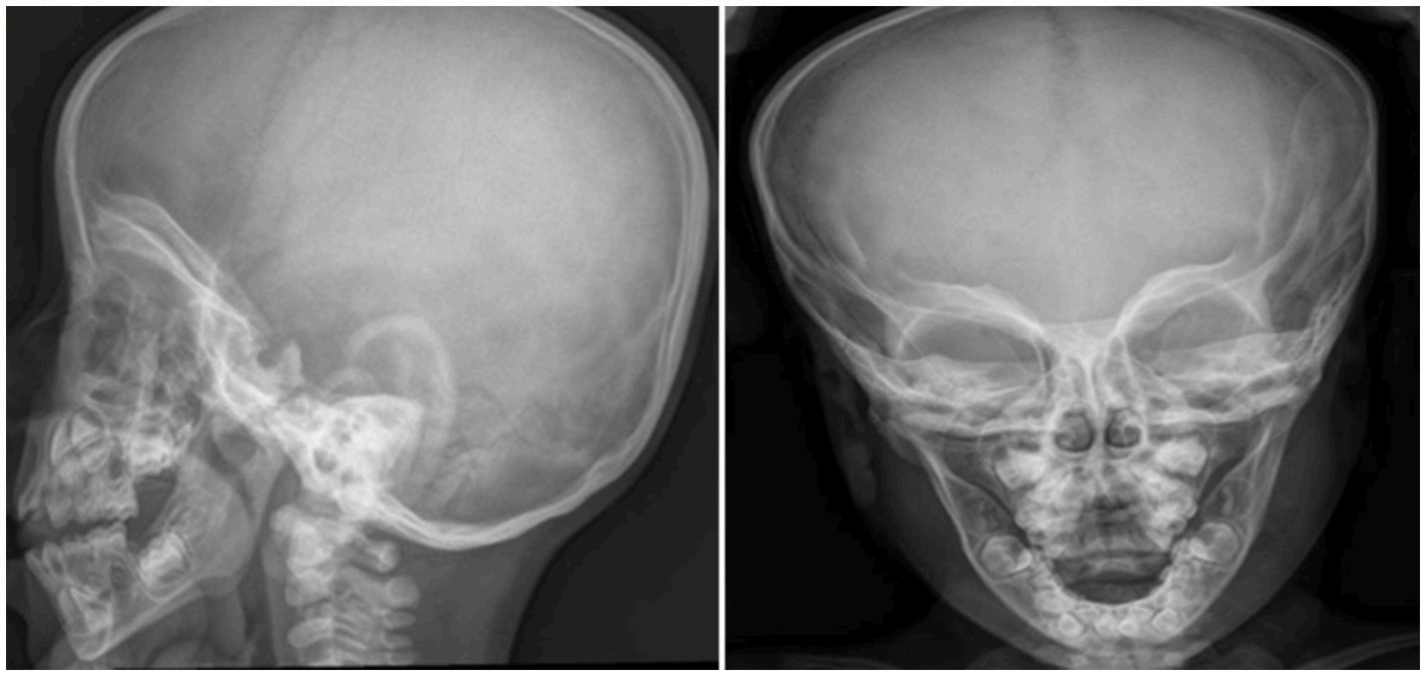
Cranial x-ray shows increased biparietal diameter, frontal bossing, “harlequin mask apparence” of orbits with increased density and thickness of the orbital roofs.
